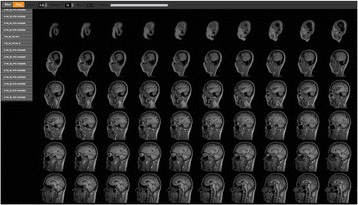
A few sagittal MRI images displayed in titled mode after loading approximately 1,500 DICOM files from 11 MRI scans. It took approximately ten seconds to load all the DICOM files into NiftyView. The images are organized into different vertical tabs by the sequence names stored in the DICOM files
